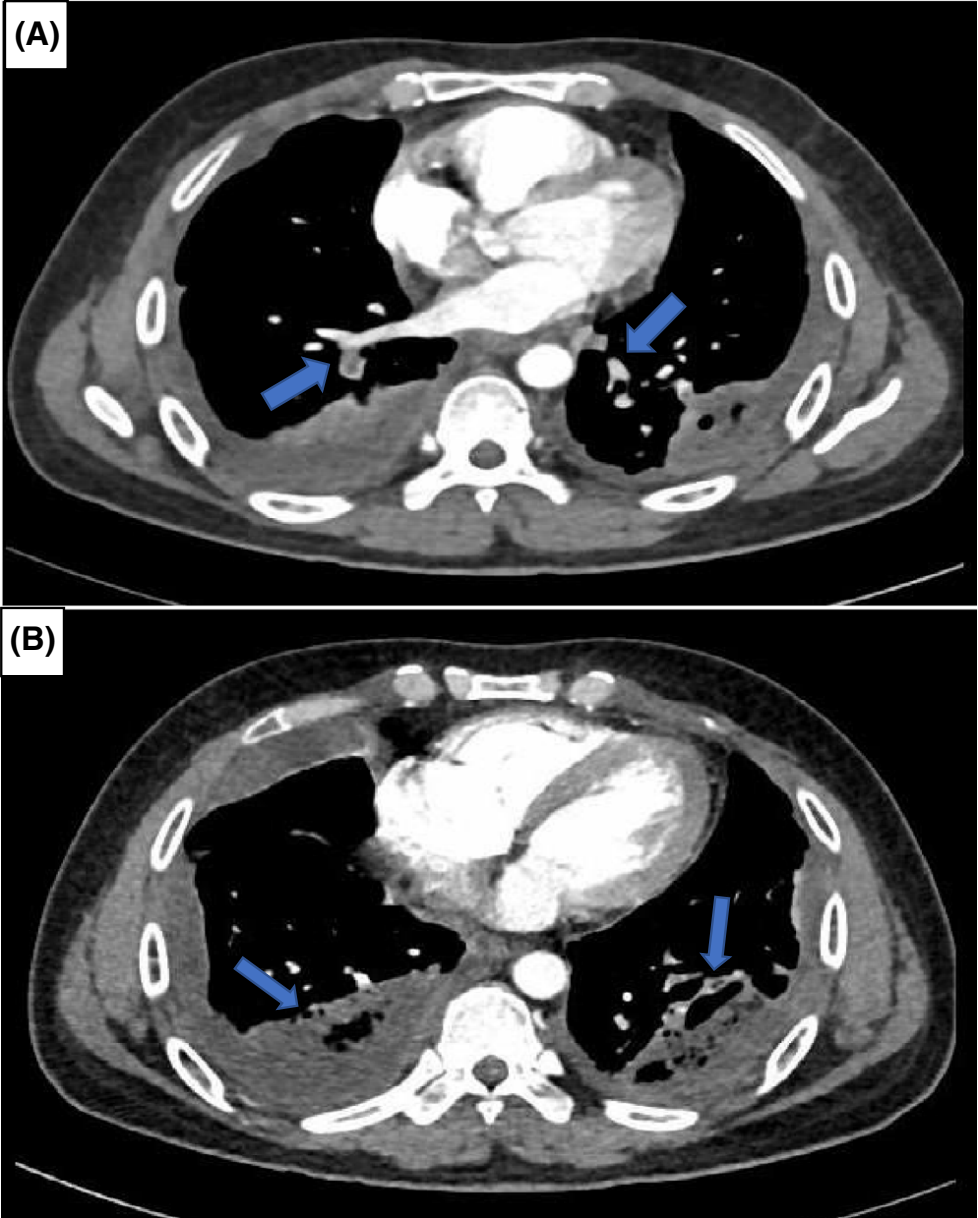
Computed tomographic pulmonary angiography (CTPA) images. (A) Showing bilateral segmental pulmonary emboli. (B) Revealing bilateral lower lobe consolidates with air bronchograms and pleural effusion
